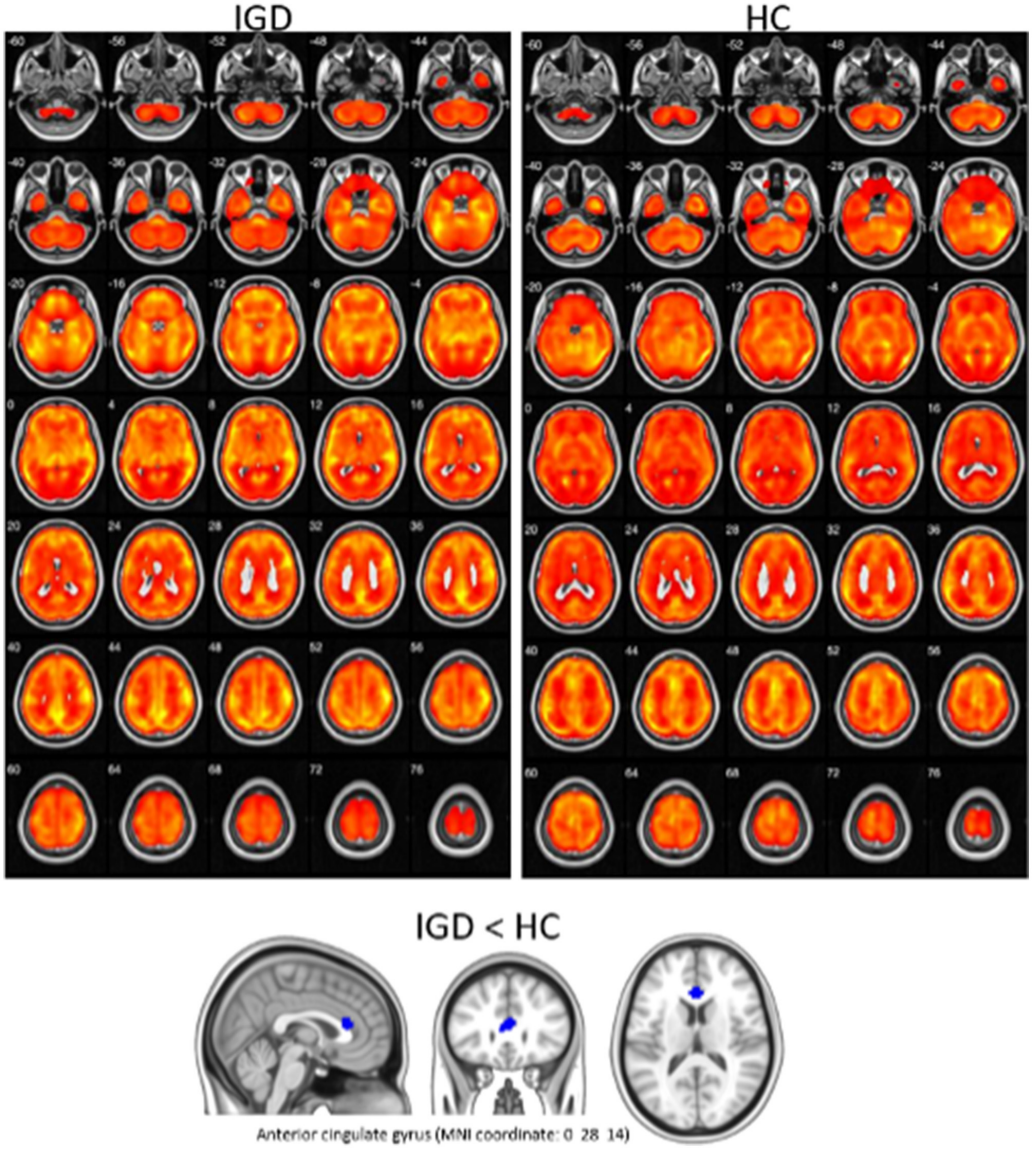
Visualization of mean metabolic uptake of ^18F^-FDG-PET in IGD and HC. Top: areas showing significant glucose metabolism in both IGD and HC, using one-sample t-test (corrected *p* < 0.05, cluster size (*k*) > 100). Bottom: IGD showed lower glucose metabolism in anterior cingulate gyrus, compared with HC (*p* < 0.005 uncorrected, *k* > 100).
